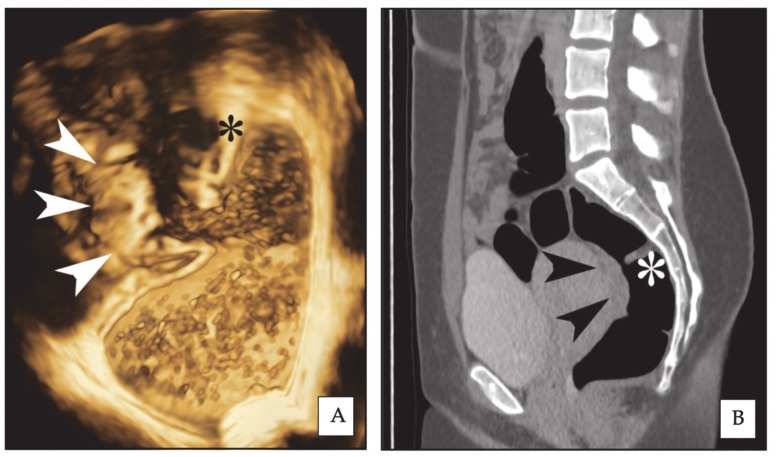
Same rectal endometriotic nodule (arrowheads) is shown in three-dimensional rectal water contrast transvaginal ultrasonography (3D-RWC-TVS) (A) and computed tomographic colonography (CTC) (B, sagittal plane). The asterisk indicates the same rectal Houston’s valve. The nodule has largest diameter of 2.6 cm.
